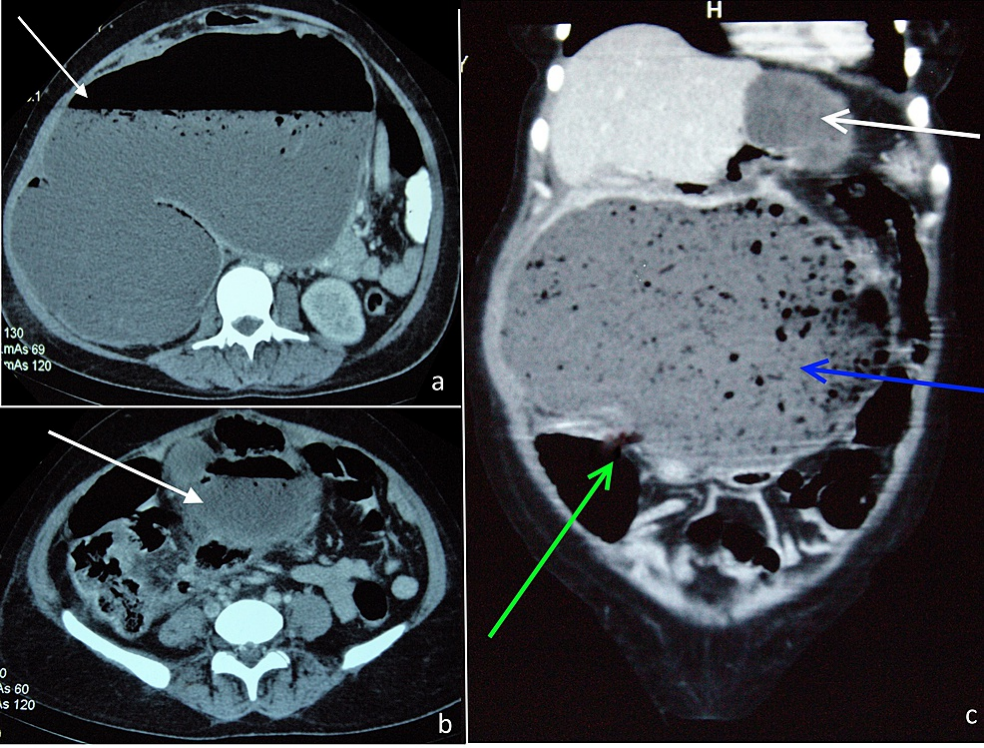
CECT showing multiple hydatid cyst in the (a) liver and (b) omentum (white arrow). (c) Coronal view showing large hydatid cyst from liver (blue arrow) ruptured into the colon (green arrow). CECT - Contrast-enhanced computed tomography
